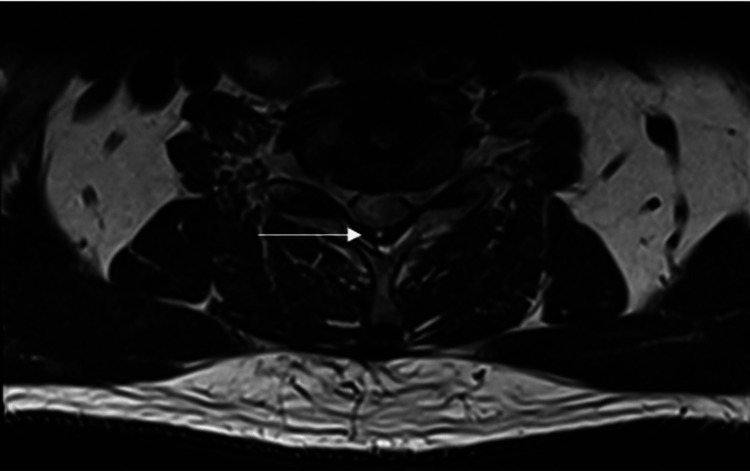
Postoperative T2-weighted MRI of the cervical spine (axial view) New focal gliosis/edema within the spinal cord at C6/C7 (arrow) MRI: magnetic resonance imaging
